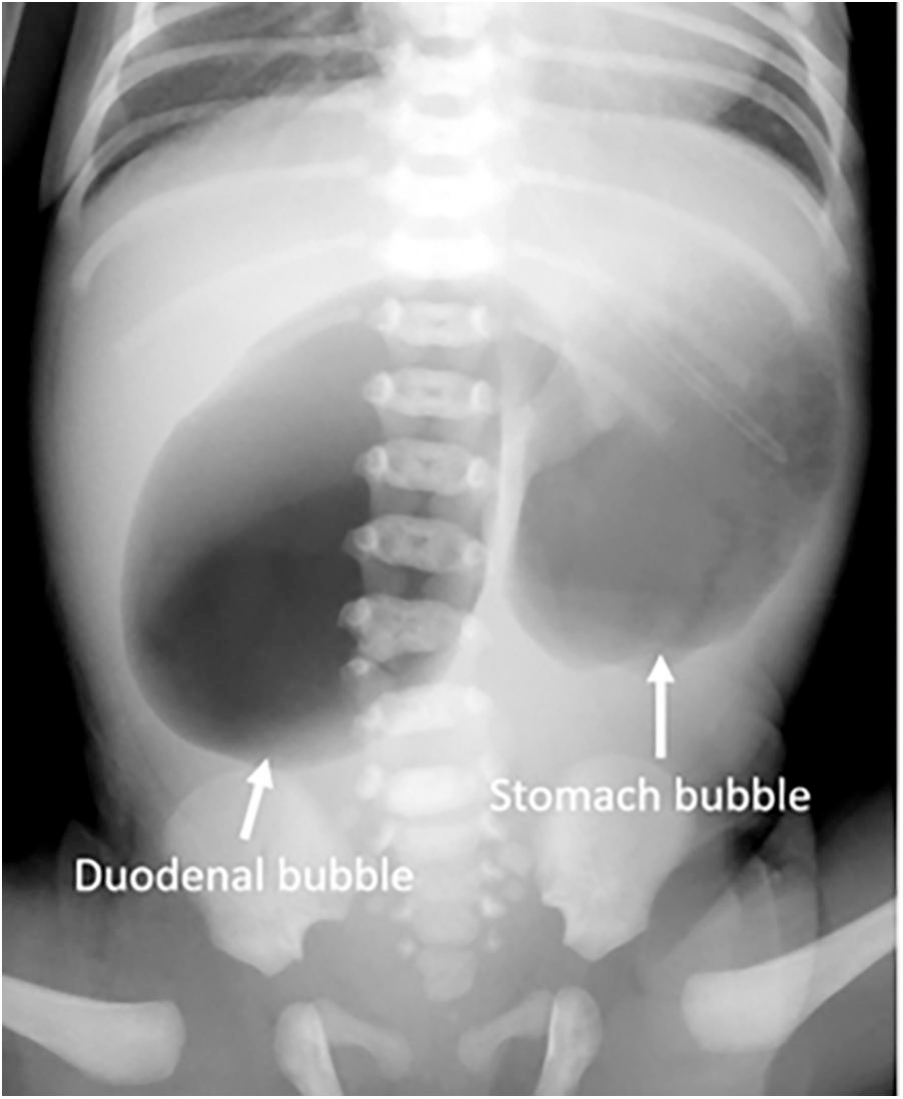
Preoperative x-ray shows the “double bubble” sign with no gas in the distal bowel (arrow).
